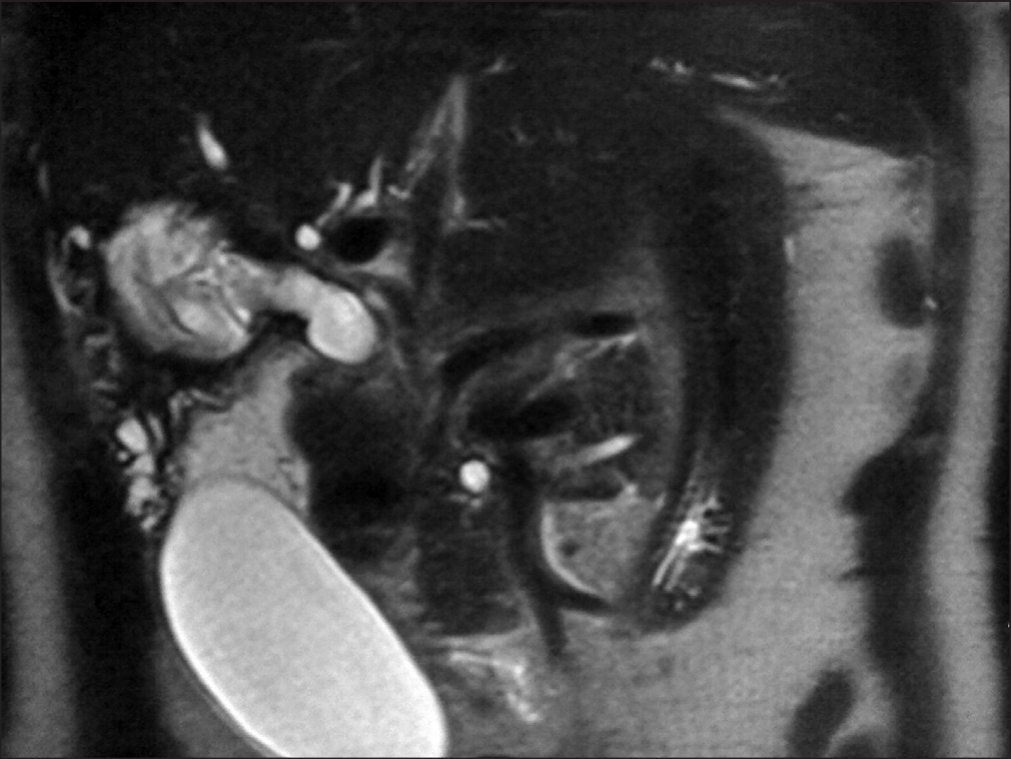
Coronal T2W MR image shows a definite communication of the liver cyst with the right posterior sectoral duct
